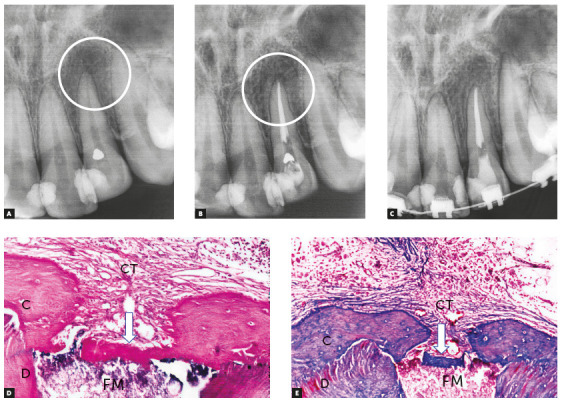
Chronic periapical lesion compatible with incipient immunogenic periapical granuloma (circle) associated with pulp necrosis and canal contamination. Tooth was moved orthodontically soon after that. In some weeks, periodontal ligament was repaired and restored with new connective tissue (CT); cementoid tissue may form at interface (arrows) depending on type of filling material (FM). (D=dentin, C=cementum - HE and MT, 10X. (Source: Esberard^9^, 1992).
